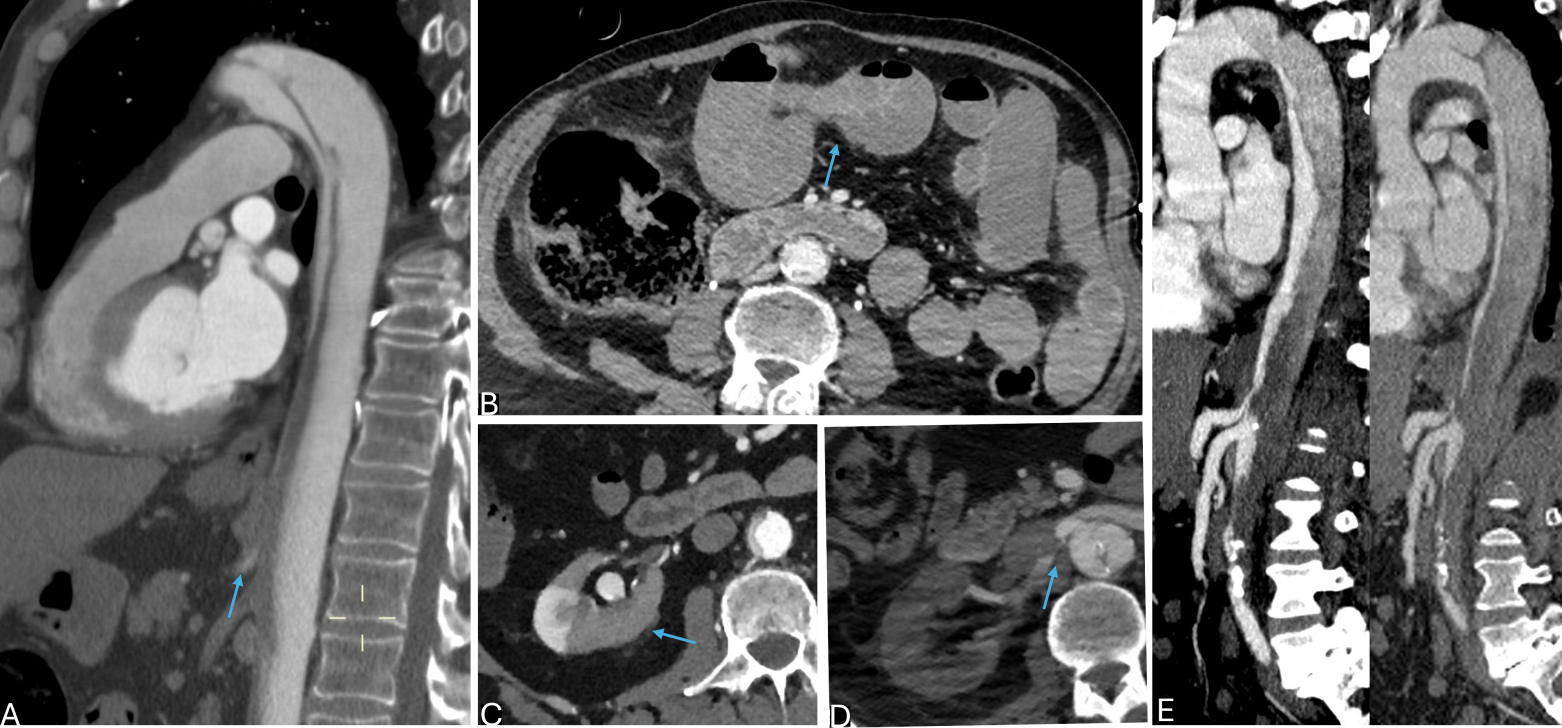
Complicated type B aortic dissection (TBAD) at CTA: vascular and visceral malperfusion.
